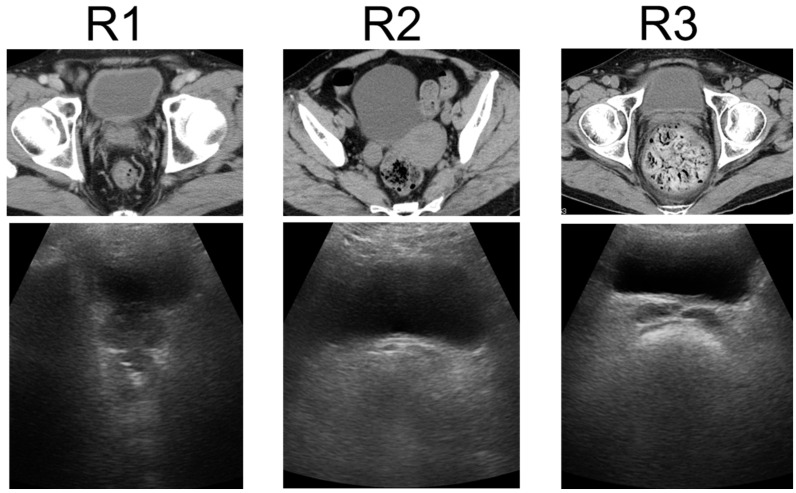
Ultrasonographic rectal findings. Transverse US images show no FR or gas in the rectum as no reflection, FR as a half-moon-shaped moderate reflection with an acoustic shadow, and hard FR as a crescent-shaped strong reflection with an acoustic shadow. US findings of the rectum were classified as R1 (no FR), R2 (FR), or R3 (hard FR). US: ultrasonography, FR: fecal retention.
